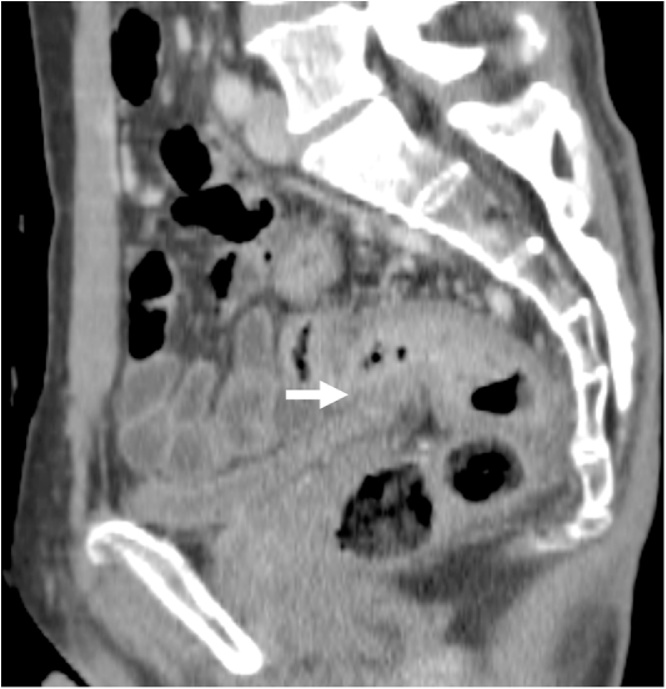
Abdominal CT after chemotherapy revealed that the tumor size was markedly decreased and extensive invasion between the rectal tumor and bladder wall was not observed (arrow). CT, computed tomography.
